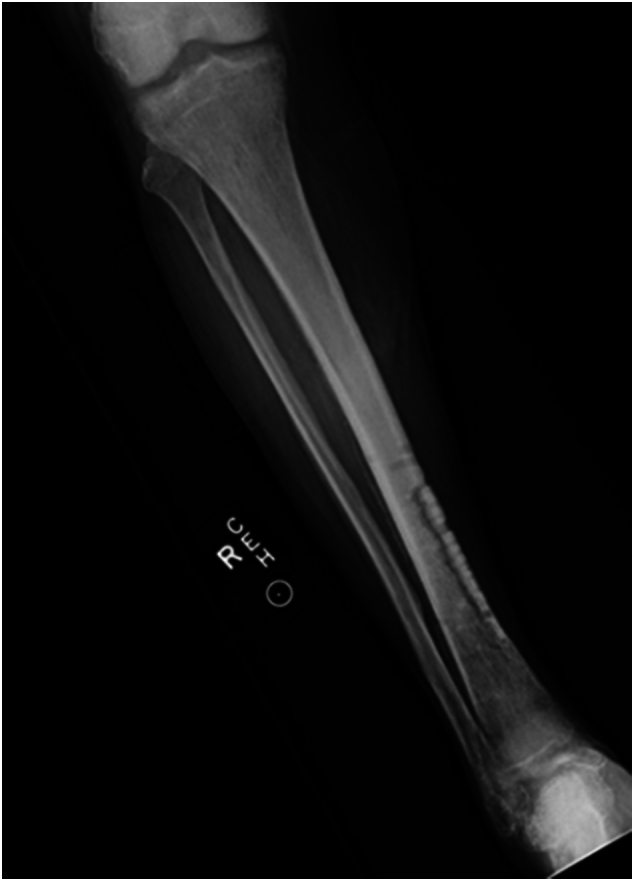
Final radiographs demonstrating healed bone transport segment.
